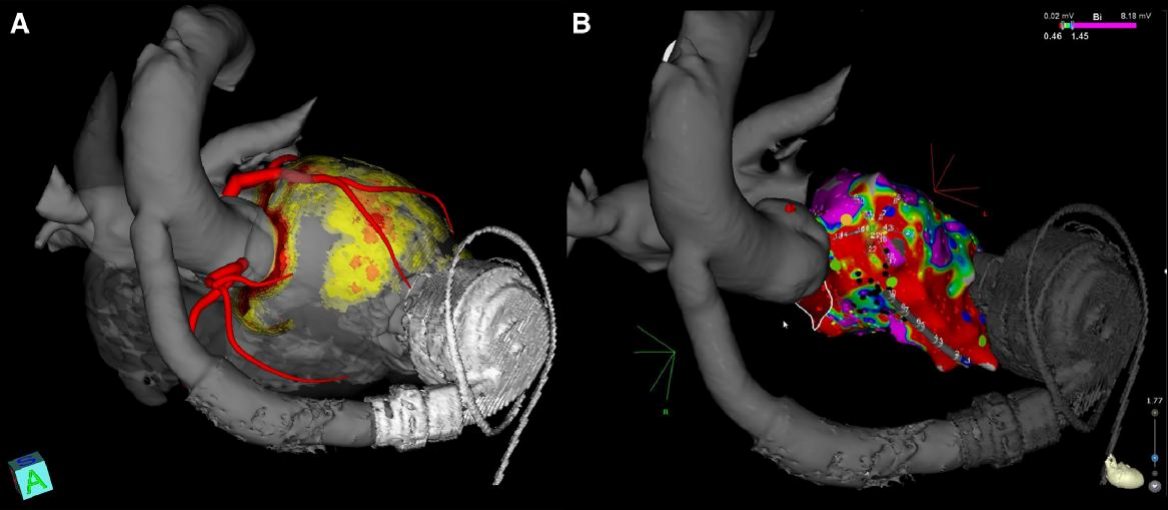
(A) CT scan of a patient with HeartMate 3 and ischaemic cardiomyopathy treated with dedicated software to be integrated into a 3D mapping system. (B) Integration of the images in the 3D mapping system with the bipolar voltage map.
